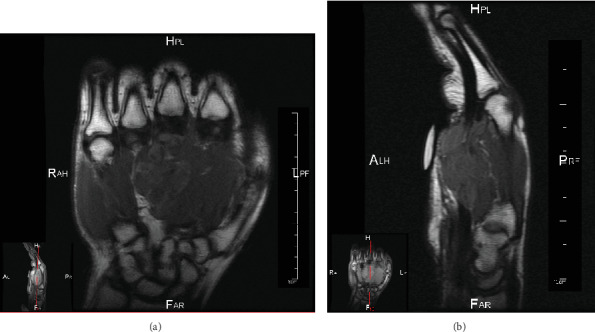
T1-weighted coronal and sagittal MRI revealing a large, lobulated, soft tissue lesion with aggressive features located on the ventral surface of the hand and extending from the mid-third digit to the fifth digit. (a) Coronal view demonstrating a hypointense enhancing lobular lesion at the third ray. (b) Sagittal view showing a soft tissue lesion surrounding the flexor tendons and extending into the interspace between the head of the third and fourth metacarpals.
